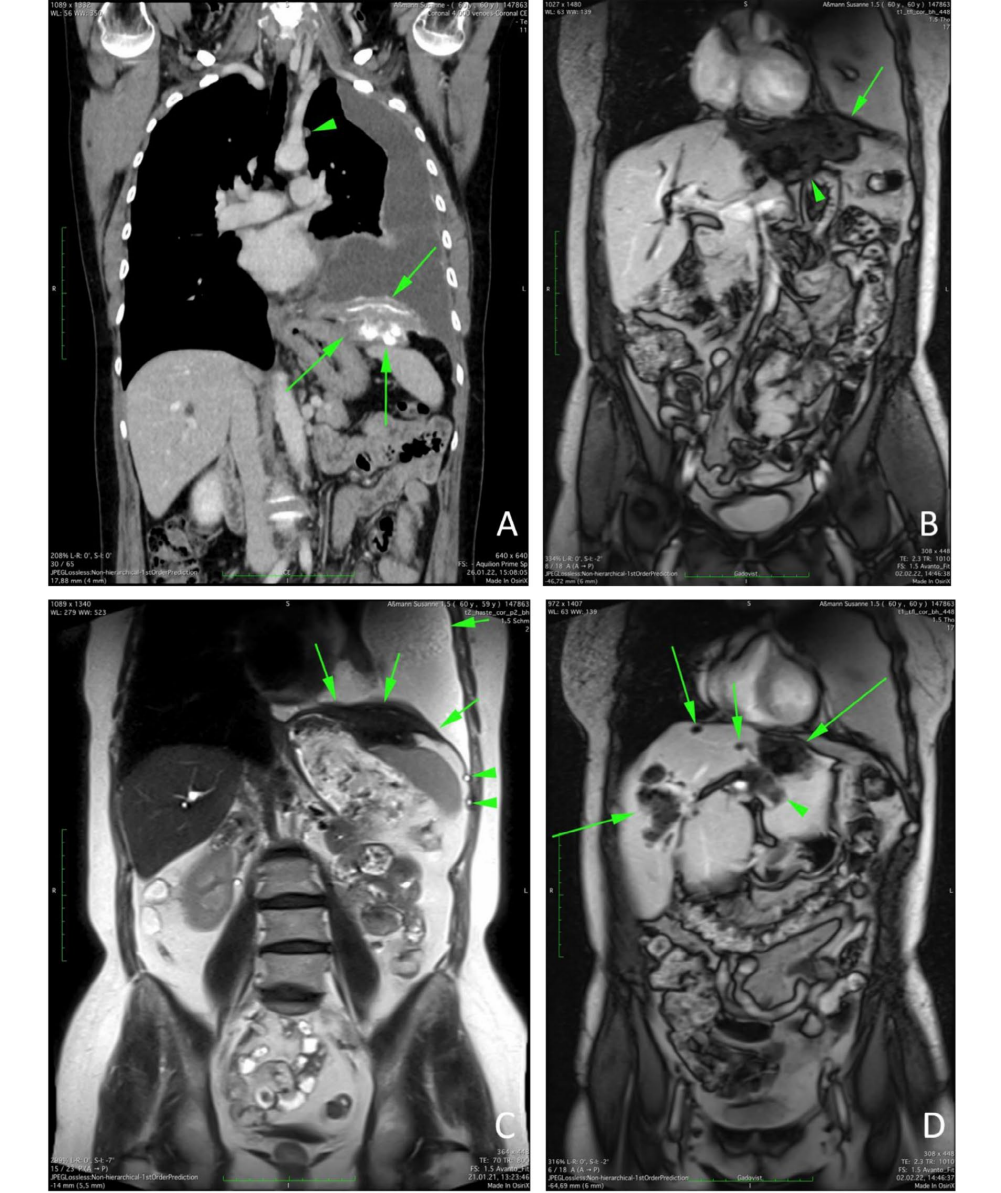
A: CT scan, post contrast, coronal plane. Green arrow: fibrotic and calcified infiltration of the left diaphragm and the spleen and pleural effusion left due to *Echinococcus multilocularis*. Green triangle: echinococcal cyst in the left pleura. B: MRI scan, T1 weighted, late phase, post contrast, coronal plane. Green arrow and triangle: per continuitatem inflammatory hypovascular/ fibrotic infiltration due to *Echinococccus multilocularis* from the liver towards the left diaphragm. C: MRI scan, T2 weighted, coronal plane. Green arrows: fibrotic infiltration of the left diaphragm and the spleen with pleural effusion left due to *Echinococcus multilocularis*. Green triangle: small echinococcal cyst in the left pleura. D: MRI scan, T1 weighted, late phase post contrast, coronal plane. Green arrows: confluent echinococcal cysts and confluent hypovascular fibrotic infiltrations in both liver lobes. Green triangle: perihilar fibrotic inflammatory infiltration.
